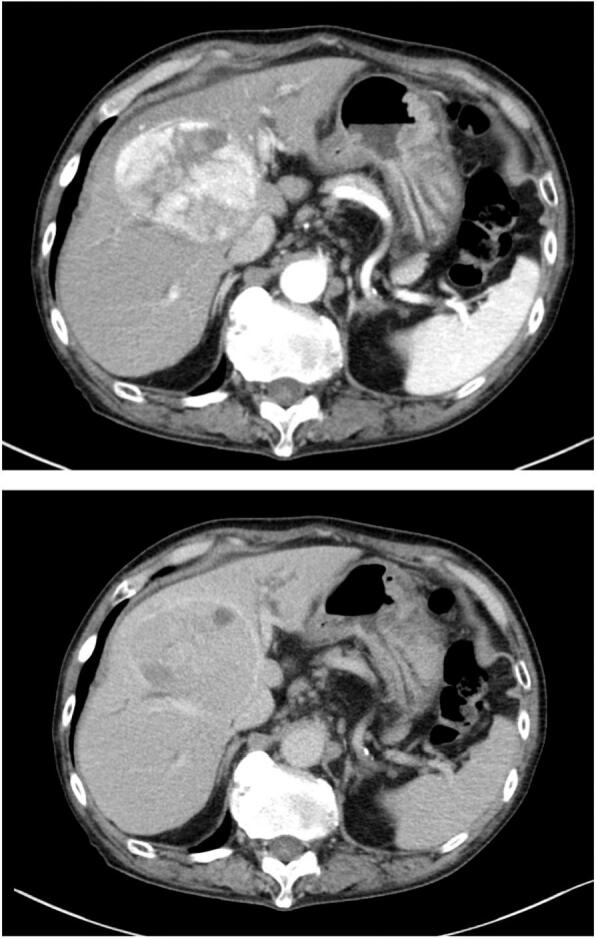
Contrast-enhanced CT shows a 76 mm in diameter tumor. Contrast-enhanced CT reveals a 76 mm diameter tumor with heterogenous enhancement on the arterial phase and a wash-out pattern on the portal phase in the right anterior segment.
